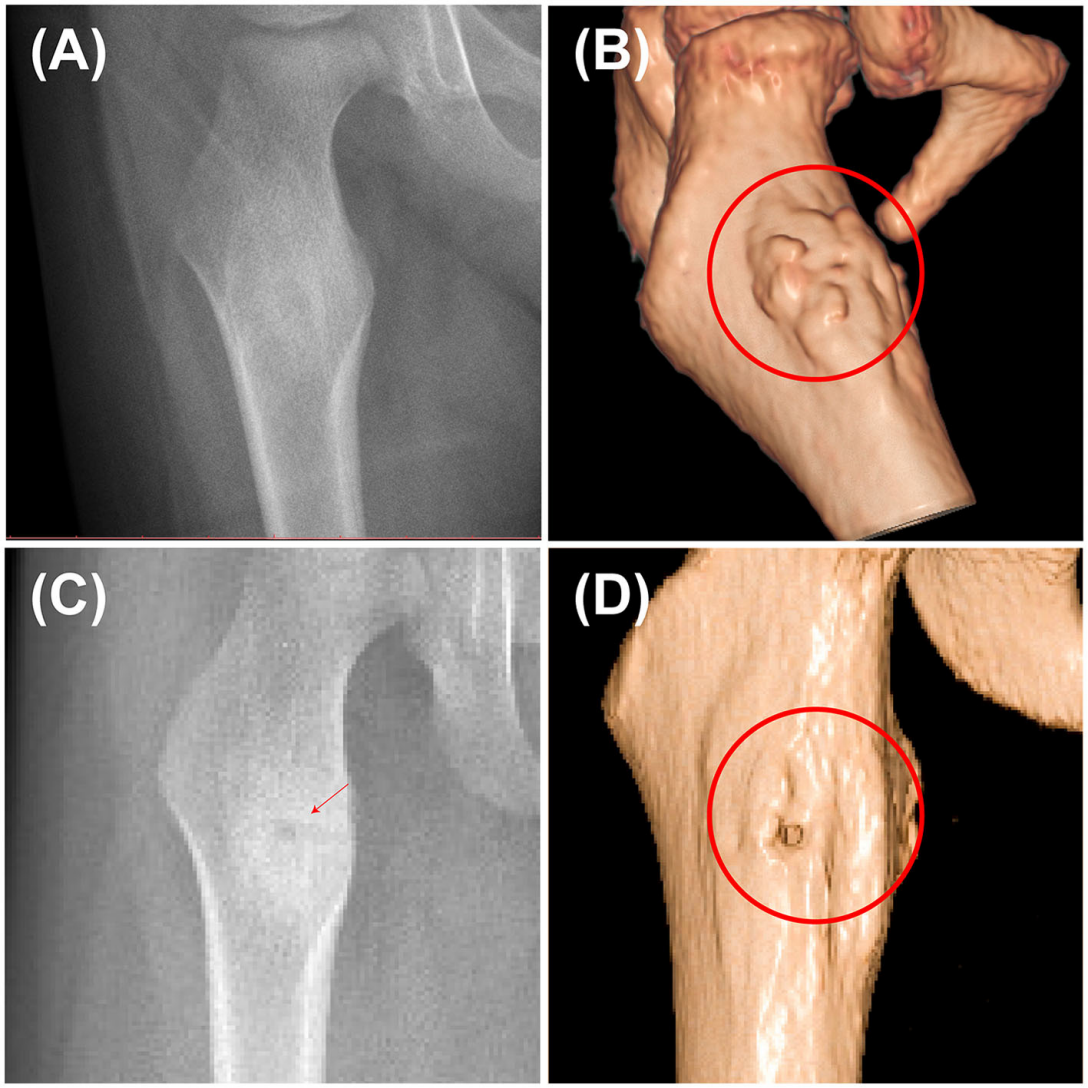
Pre-operative and post-operative radiographs of a 3-year-old child confirmed with OO using the robot-assisted surgery. (A) Pre-operative X-ray image of the nidus. (B) Pre-operative 3D reconstruction image showing the thickening of the nidus. (C) Post-operative X-ray image at 3rd month. (D) Post-operative 3D reconstruction at 3rd month showing that the nidus greatly shrank (red arrow and cycle, the nidus).
